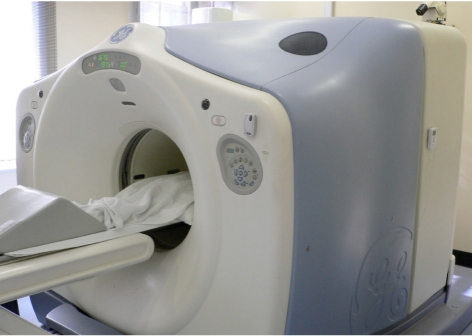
The Discovery LS (GE Medical Systems, Milwaukee, WI) was the first commercial PET-CT scanner to become available. This scanner at these authors’ institution was installed in 2001 and has now performed over 12,000 scans. Implementation of this scanner involved end-by-end installation of a 4-slice Lightspeed plus CT with the GE Advance NXi PET scanner with a new patient bed. The CT and PET components operated on independent computer platform.
